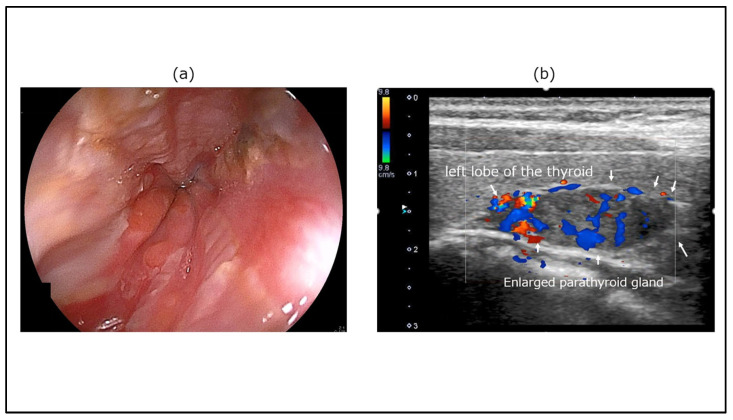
A case of secondary constipation in the context of endocrine disorders. (a) Upper gastrointestinal endoscopy image. Mucosal damage was observed in the esophagus. (b) TUS image (Aplio i700 TUS-I700; Canon Medical Systems, Otawara, Japan). Ultrasonography revealed an enlarged parathyroid mass with abundant blood flow signals in the dorsal thyroid gland. The enlarged parathyroid gland is indicated by arrows.
